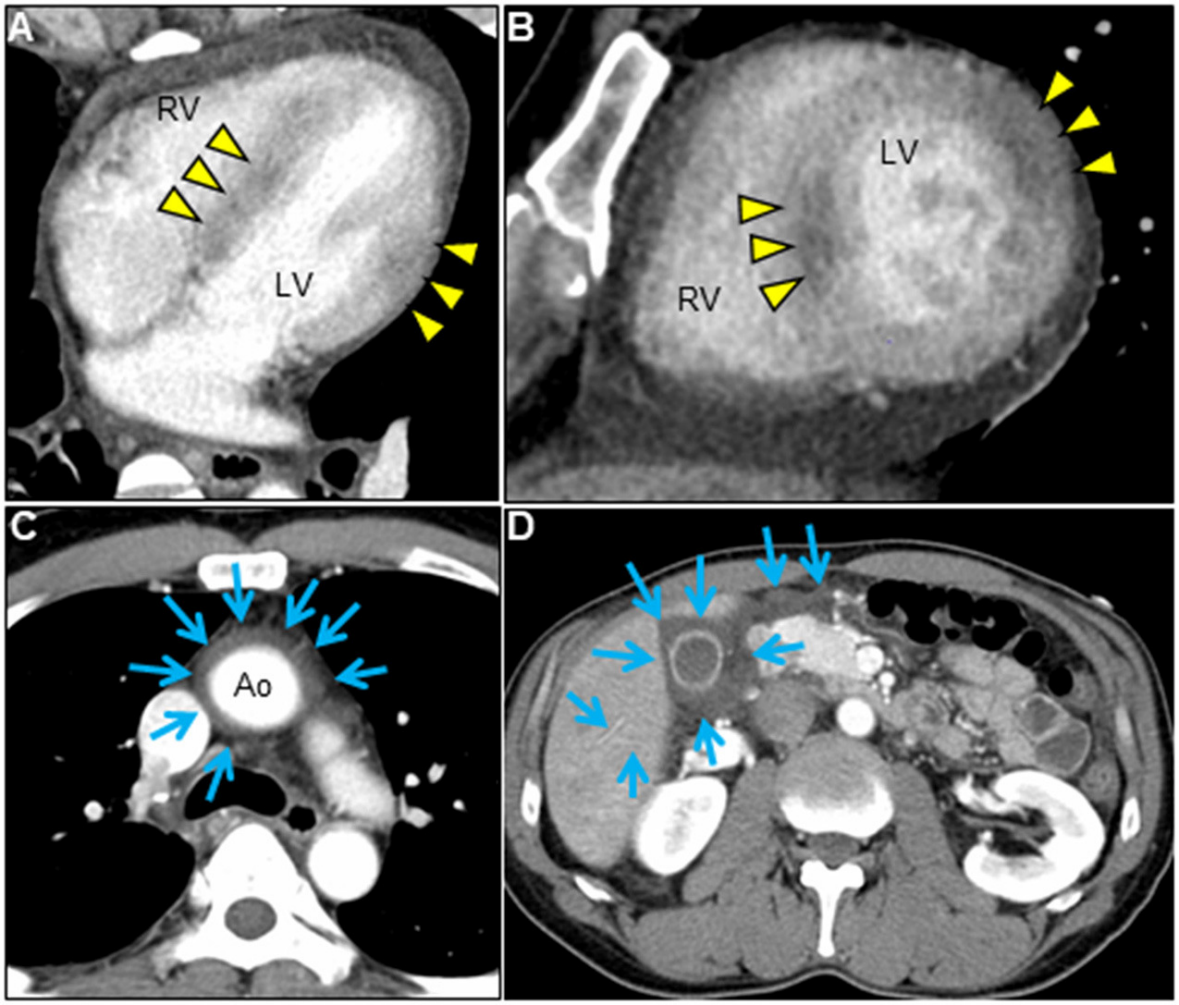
Chest/abdominal contrast-enhanced CT images. Axial (A,C,D) and sagittal (B) views. Contrast-enhanced CT reveals LV wall thickening with pericardial effusion, decreased myocardial enhancement of both mid-layer in the basal-to-mid septal wall and transmural myocardium in the mid anterolateral wall (A,B, arrowheads), and fluid accumulation in pericardial sinuses (C, arrows). Airless low-attenuating areas in the region of gallbladder wall thickening and around the hepatic round ligament and periportal space, indicating marked systemic edema (D, arrows). CT, computed tomography; Ao, aorta; LV, left ventricle; RV, right ventricle.
